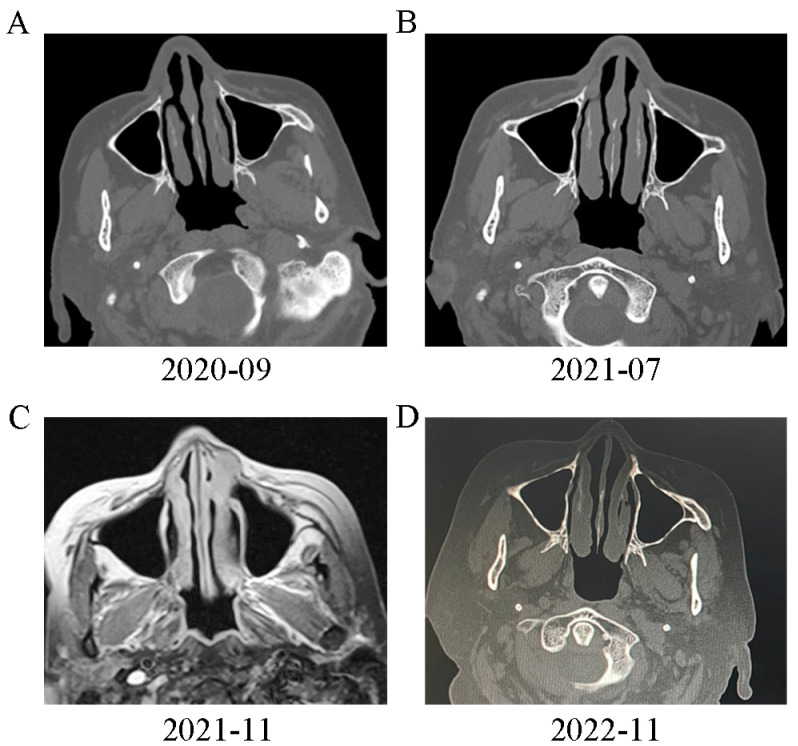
(A) Sinonasal CT in 7 September 2020 revealed irregularities in the left sinonasal bone with multiple low-density areas and the local swollen soft tissues. (B) The sinonasal CT in 29 July 2021 indicated enlargement of the destruction area in sinonasal bone with both sides affected. (C) Sinonasal magnetic resonance imaging in 5 November 2021 indicated local narrowing of the left nasal meatus in addition to the above manifestations. (D) After prednisone treatment for 3 months, sinonasal CT was performed and indicated significant improvement of destruction in the sinonasal bone.
